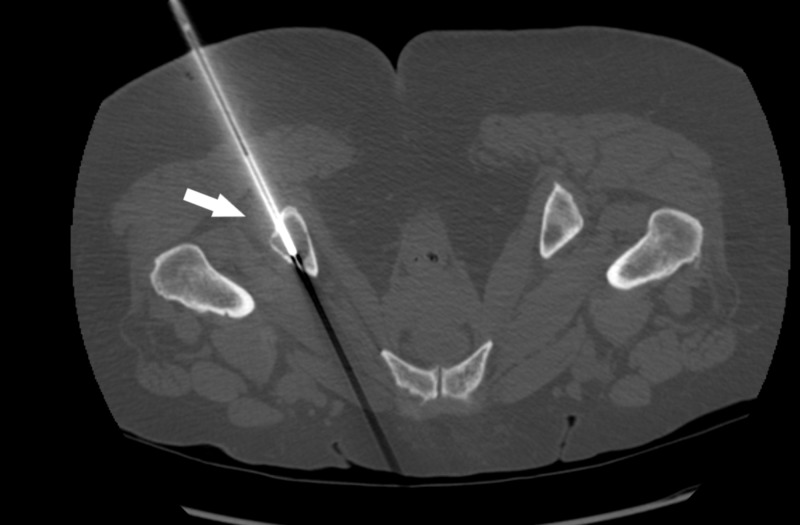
CT-guided biopsy CT-guided percutaneous biopsy of a lytic lesion in the right ischial tuberosity (white arrow).
